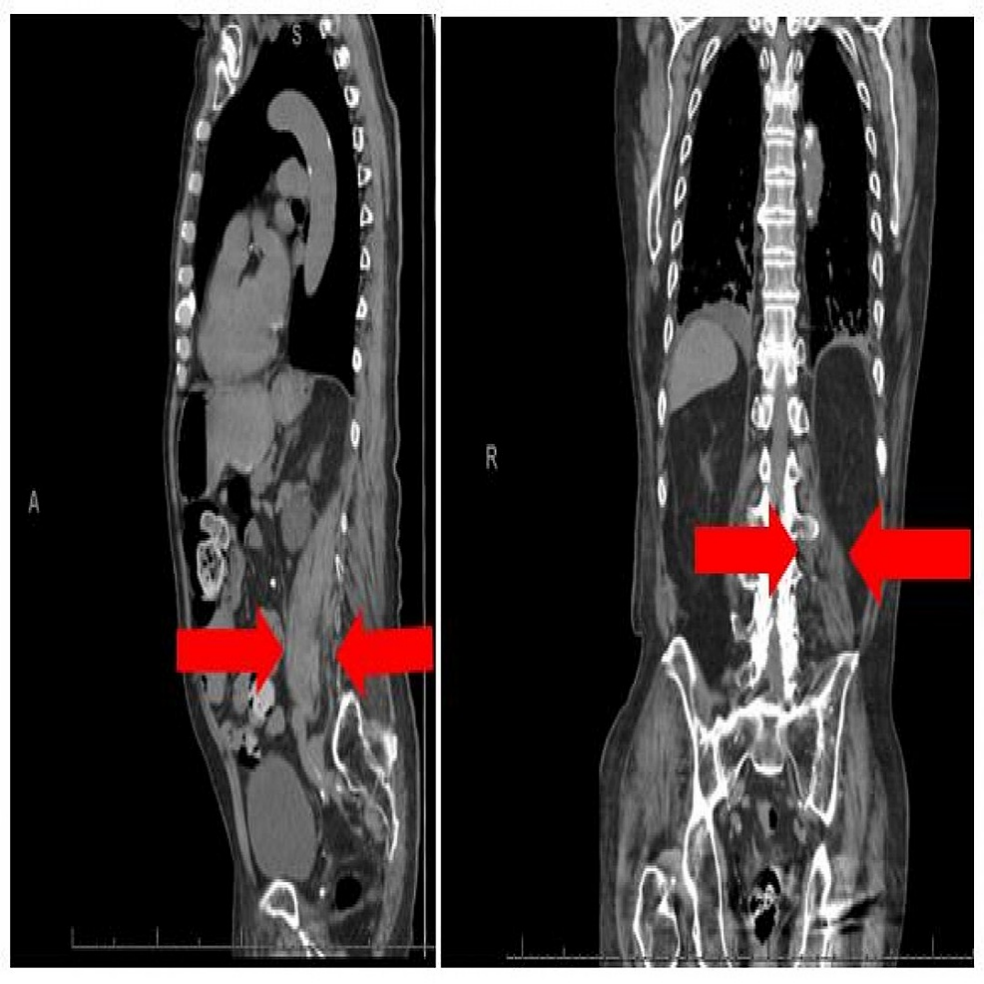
Computed tomogram (CT) with contrast; sagittal and coronal views. Red arrows show large extended left iliopsoas hematoma.
